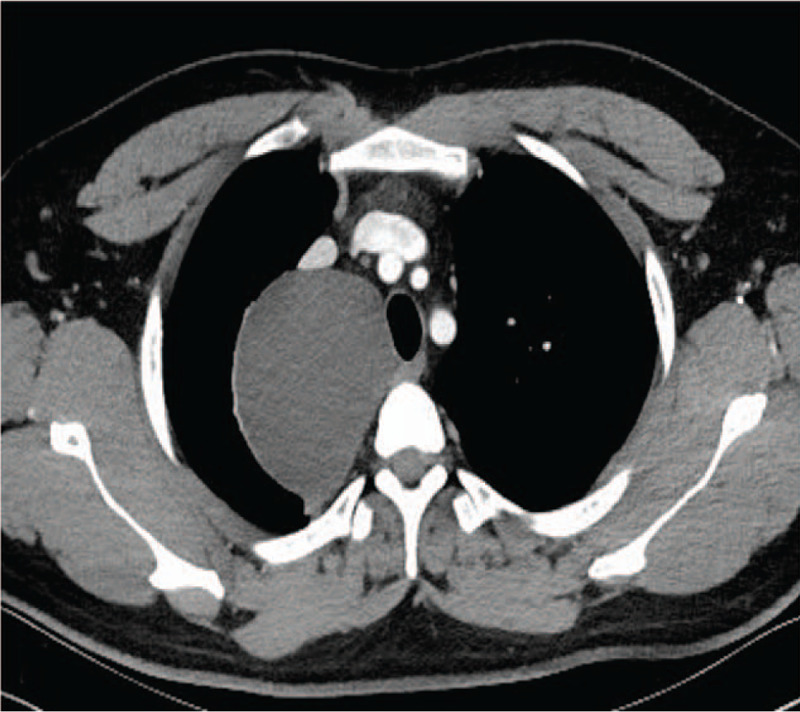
Contrast chest CT showed the enhancement of the wall of cystic lesion.
